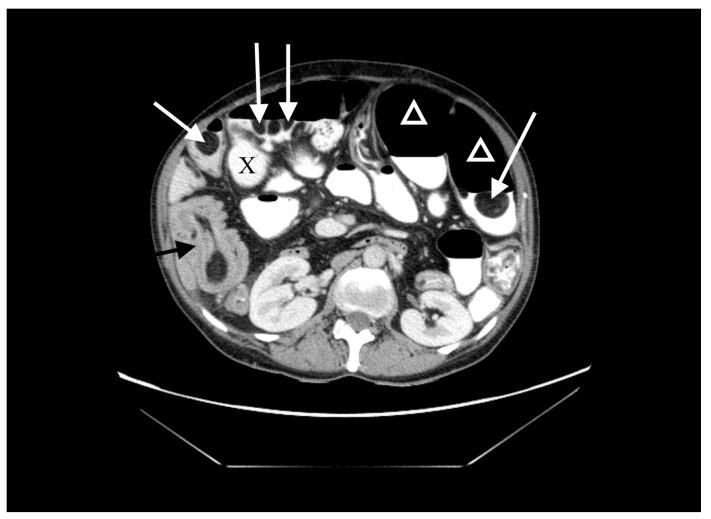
Contrast-enhanced computed tomography (CT) image of the abdomen, axial section. Multiple jejunal lipomas (white arrows), distended jejunum (triangles), one of the diverticula (X) and intussuscepted jejunum with a lipoma as the leading point (black arrow).
